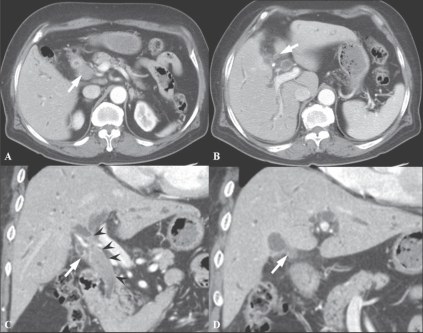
A 72-year-old man with hilar CBD cancer. Axial CT scan (A) during the arterial phase shows a soft tissue tumor in the mid-CBD (arrowheads). At the level of the right portal vein, the axial CT scan image (B) shows tumor infiltration at the orifice of the cystic duct (arrow). Oblique coronal reformation (C) reveals a soft tissue tumor extending from the hilum to the mid-CBD (arrowheads). Coronal MPR image (D) demonstrates involvement of the tumor at orifice of the cystic duct (arrow). These advantages of MPR can create a roadmap for surgeons who are not good at reading axial images
